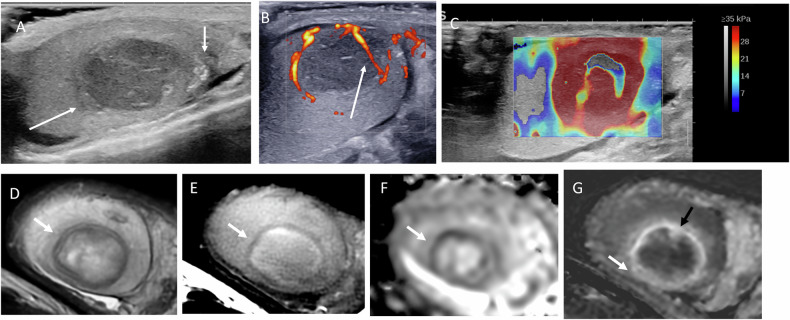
Hemorrhagic and necrotic malignant germ cell tumor presenting with acute scrotal pain. A B-mode US showing an irregular, thickened peripheral rim (long arrow) and clustered microliths (short arrow). B Power Doppler US showing a small peripheral and intralesional vessel. C SWE is demonstrating high stiffness of both the peripheral rim and central components. D T2-weighted MRI image showing a thick-walled lesion with heterogeneous signal intensity. E T1-weighted MRI image showing subtle peripheral hyperintensity suggestive of hemorrhage. F ADC map demonstrating low ADC values within the lesion wall. G Post-contrast T1-weighted MRI image with subtraction showing irregular enhancement of the wall (white arrow) and focal internal enhancement (black arrow)
